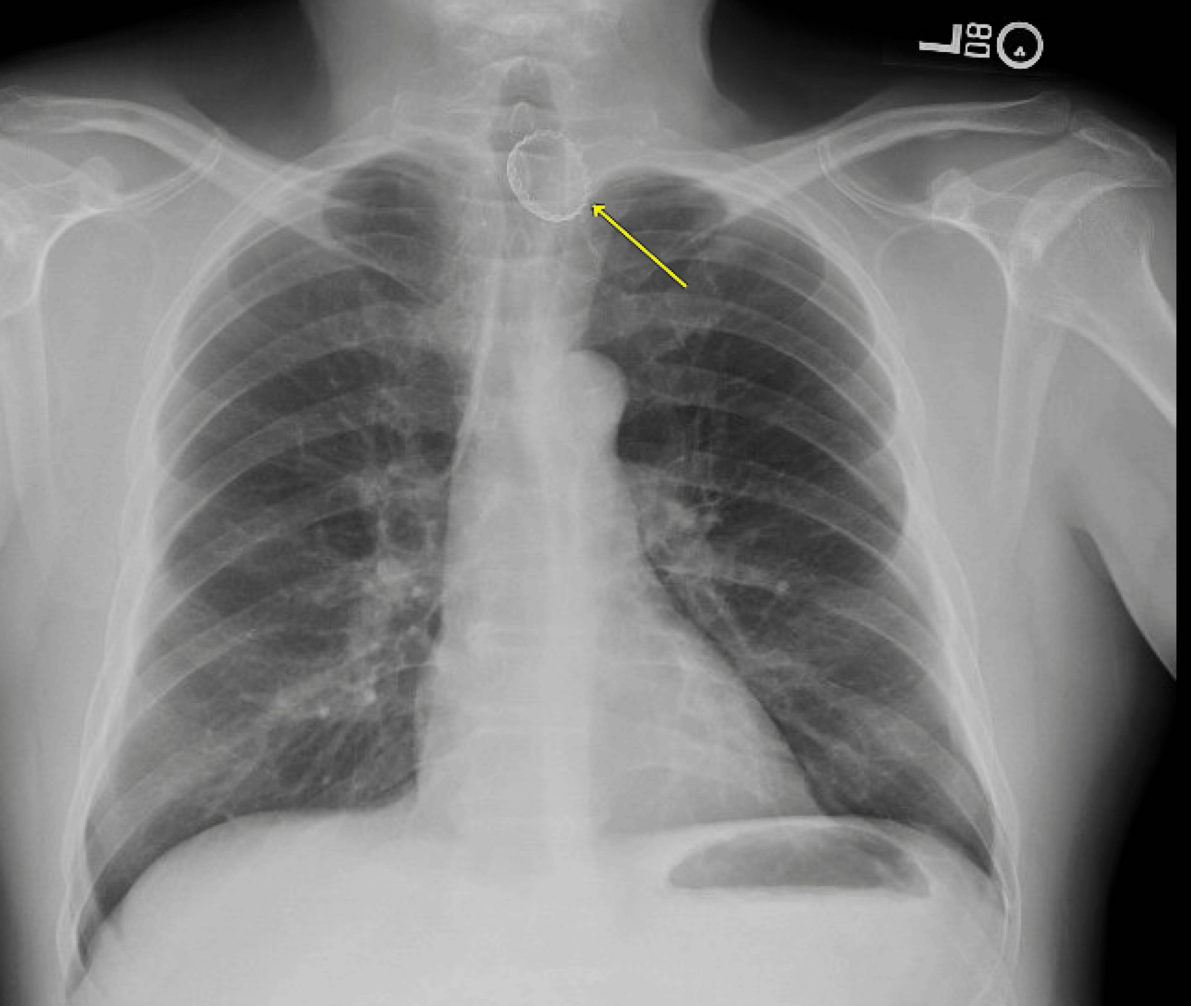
The patient's chest X-ray (posteroanterior (PA) view) A radiopaque structure projects over the upper thorax at the level of the thoracic inlet, presumably in the proximal esophagus.
